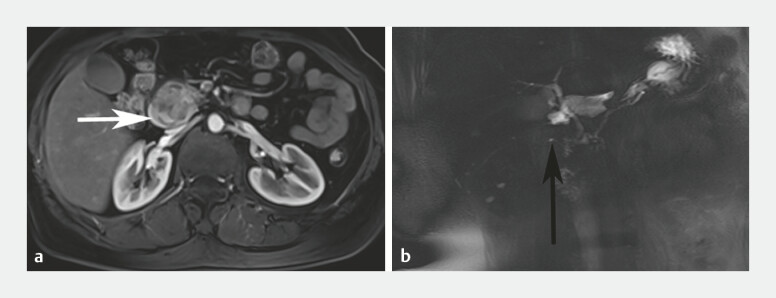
Contrast-enhanced abdominal MRI.
